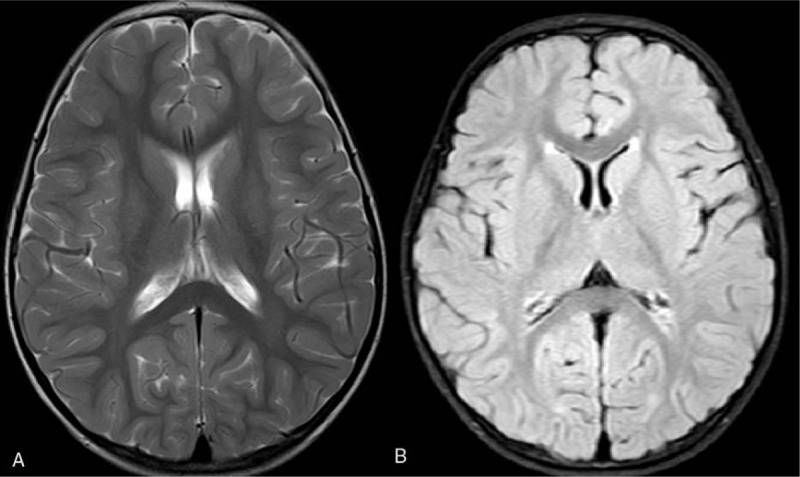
Cranial MRI showing the subdural effusion had completely absorbed on T2 (A) and FLAIR image (B).
